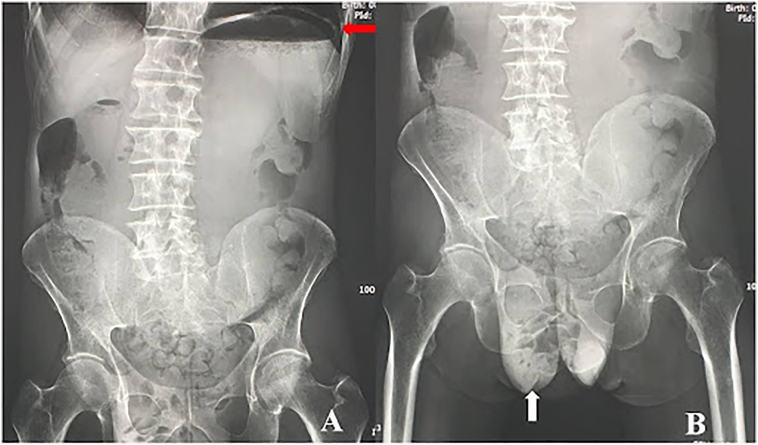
A,B. Abdominal radiography showing gastric dilatation with suspected pneumoperitoneum (Fig. 1 A, red arrow) and intestinal loops in the right inguinal hernia sac (Fig. 1 B, white arrow). (For interpretation of the references to colour in this figure legend, the reader is referred to the web version of this article.)
